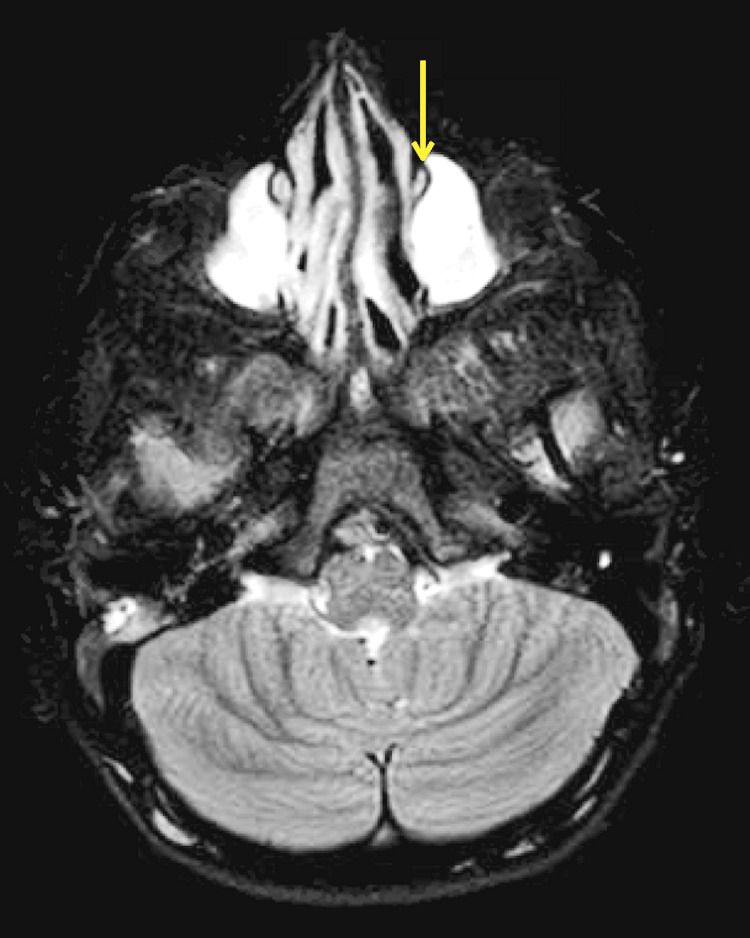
MRI STIR sequence demonstrating narrowing of nasolacrimal duct on the left side in a case with Rothmund-Thompson syndrome (arrow) MRI STIR: magnetic resonance imaging - short tau inversion recovery
